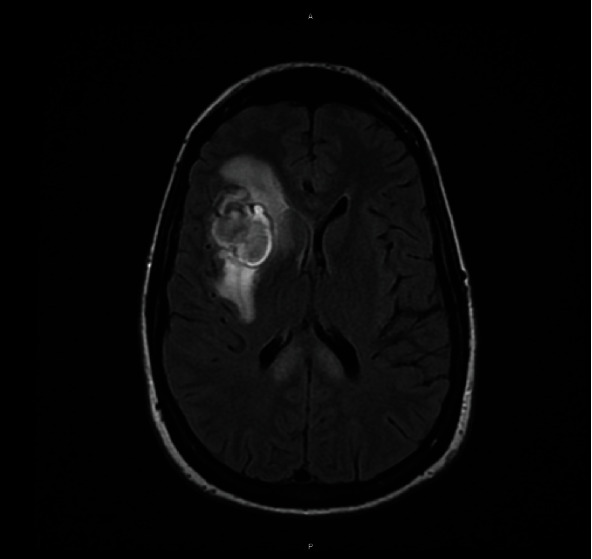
Intraparenchymal hemorrhage of the right frontal lobe on axial T2 flare MRI.
